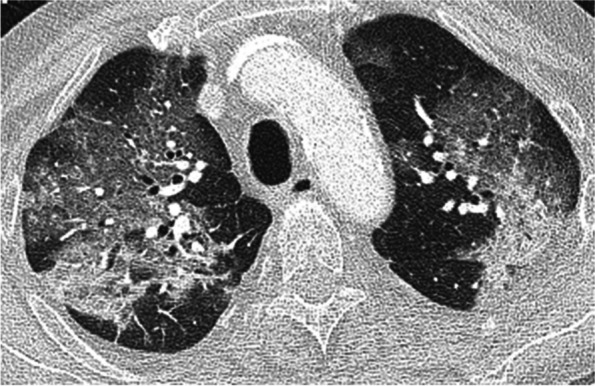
Severe Covid-19 pneumonia in a 69-year-old male with a history of lung transplants 10 years ago. Axial CT image (lung window) shows extensive bilateral patchy as well as confluent ground glass opacities. Covid-19 infection was confirmed with nasopharyngeal swab. The patient required mechanical ventilation
